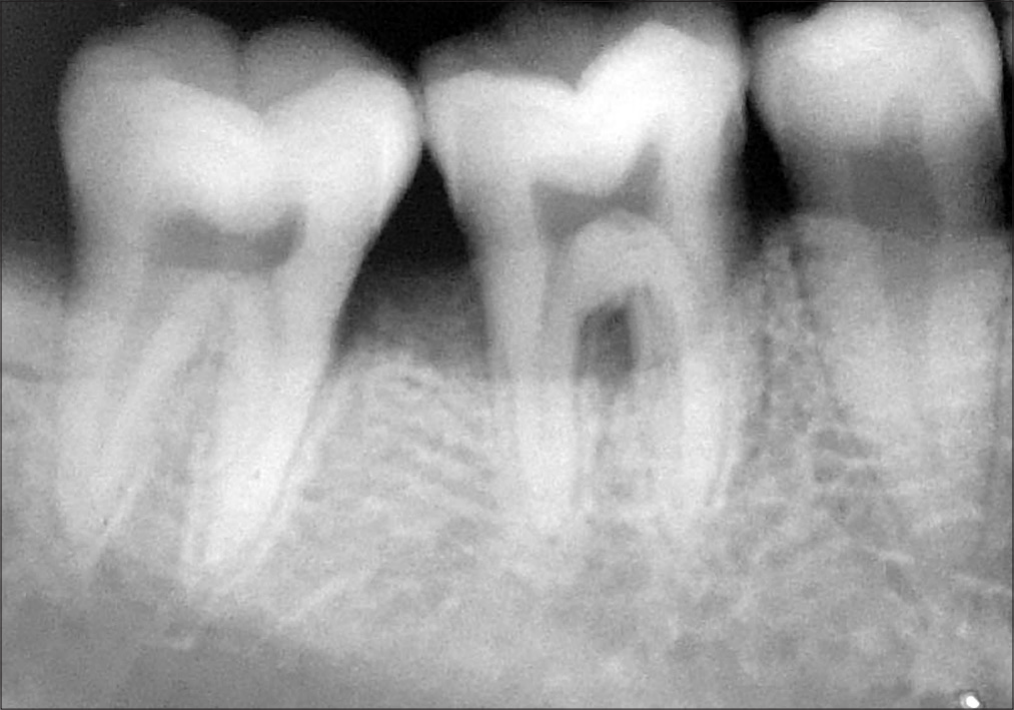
Postop radiograph (right side)
